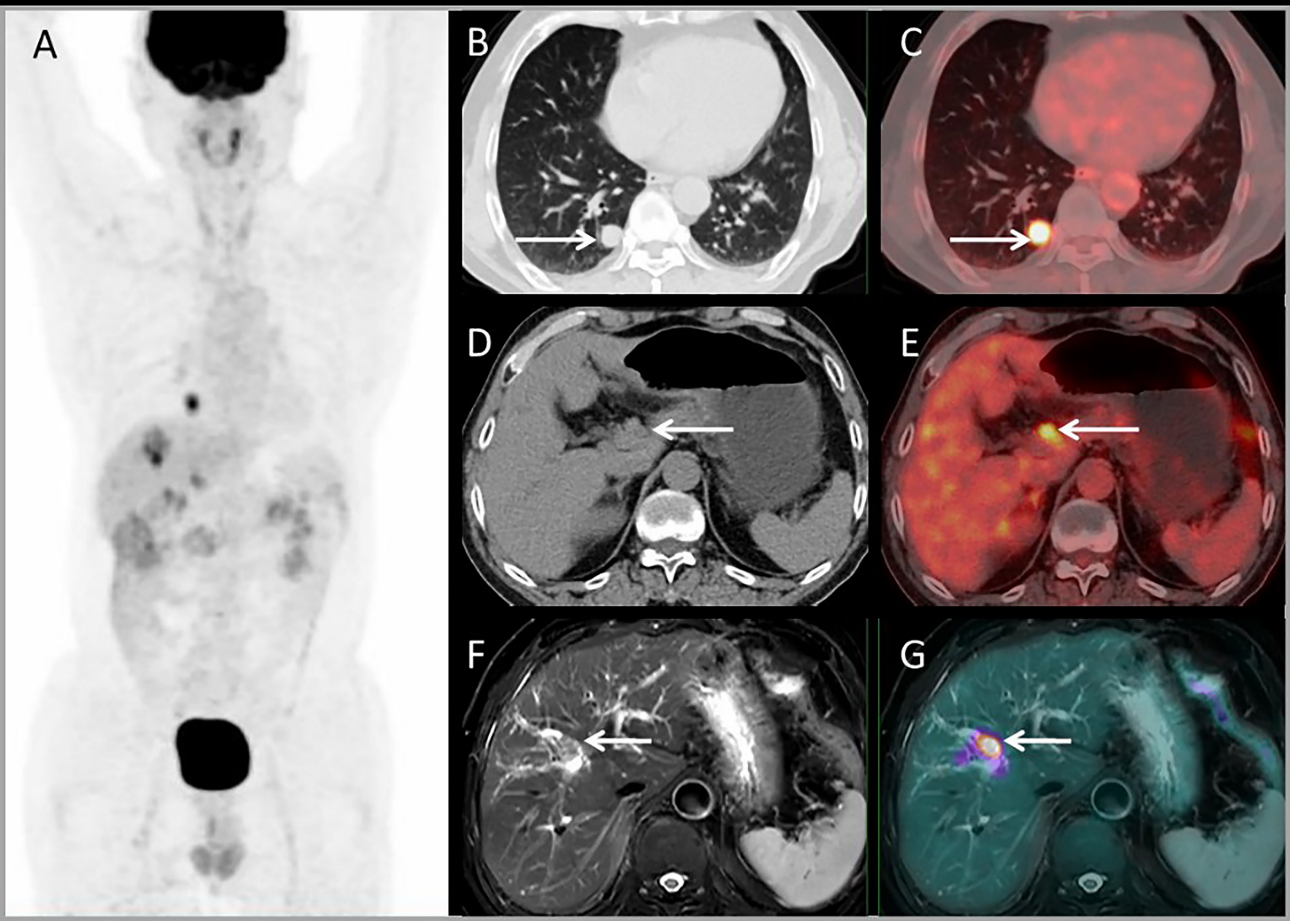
Images of a 64-year-old male with colonic liver metastasis. The MIP (A) of PET/CT showed a single lesion on the liver and EHD lesions. CT and PET/CT fusion images showed single lung metastasis (B, C) and hilar lymph node metastasis (D, E). T2WI and PET/MR fusion images showed single liver lesion with intrahepatic bile duct infiltration (F, G).
